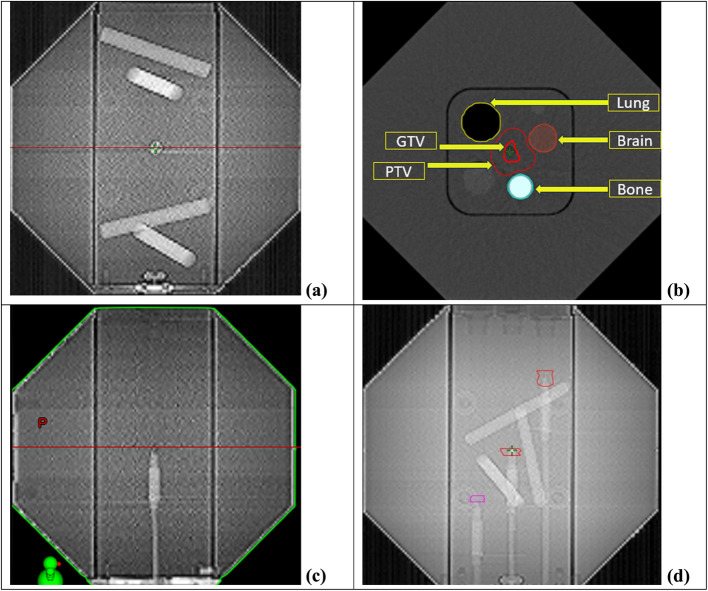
The figure represents the digitally reconstructed radiograph (DRR) of different inserts, where a shows the inserts used in CBCT, MV/KV planar dose verification and for Winston-Lutz Test, b shows the end-to-end test insert, c shows the patient-specific point dose verification with chamber, and d shows the multi-metastasis insert showing embedded ion chamber positions corresponding to three distinct planning target volumes (PTVs). The contours outlined in red, green, and magenta represent the PTVs defined for multi-target stereotactic radiotherapy. Dose measurements were performed at the geometric centers of these PTVs to ensure sampling from the high-dose region and avoid dose gradient artifacts
